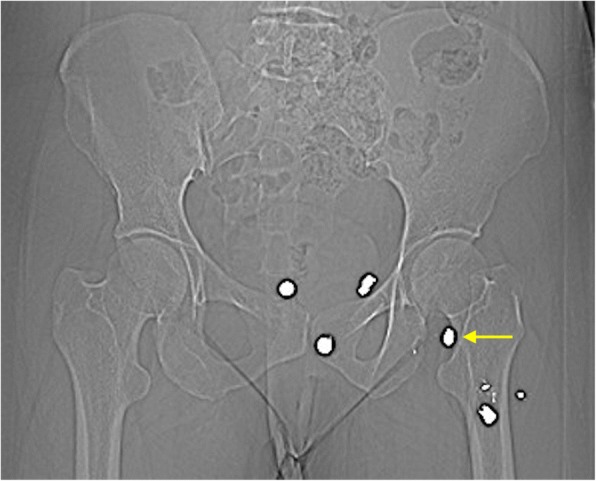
Preoperative computed tomography scan demonstrating multiple shotgun pellets in the pelvis and upper thigh area. Pellet of interest is marked with *yellow arrow*
